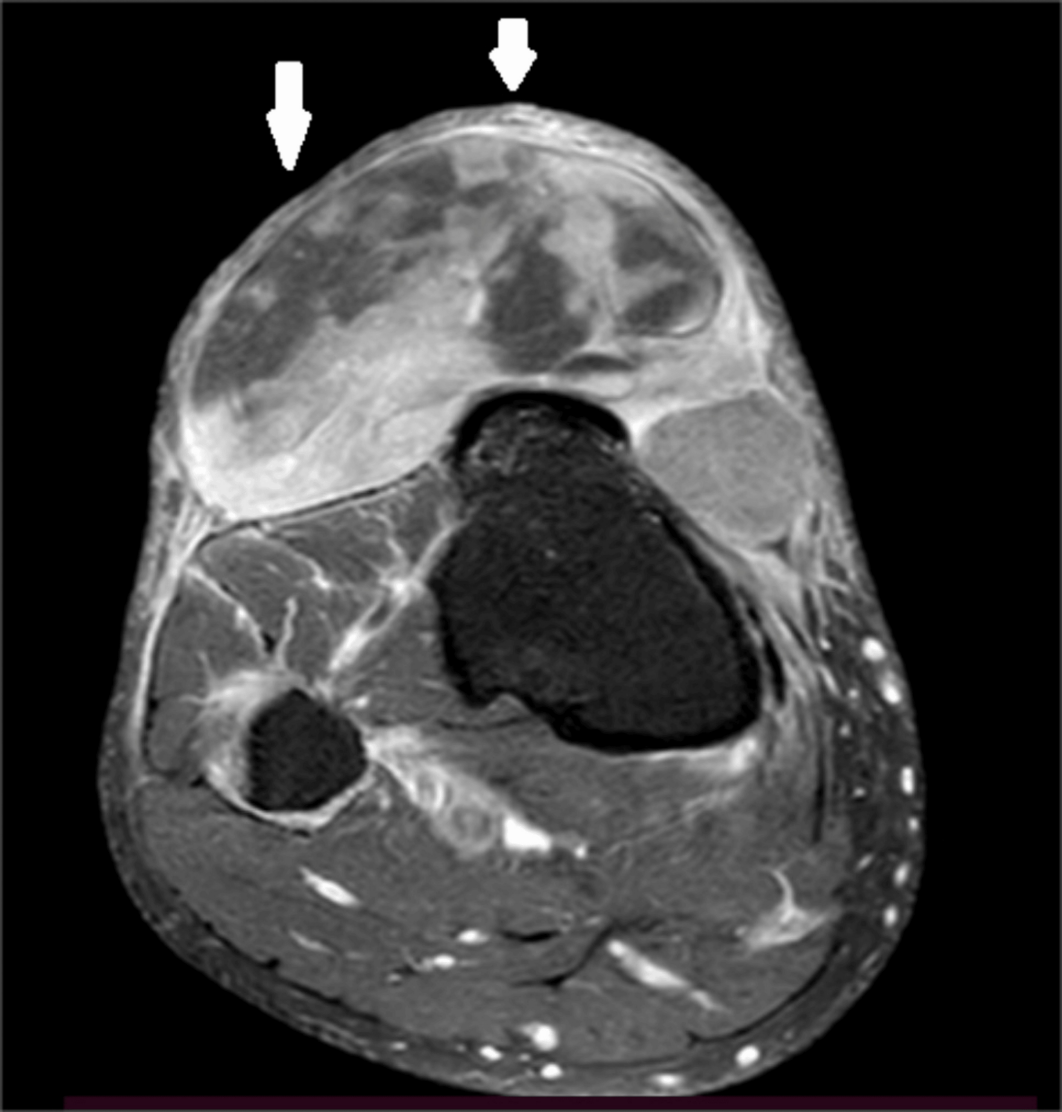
MRI image of the patient; axial section This axial section in the T2 sequence shows the multilobulated appearance of the mass, as well as the necrosis in spontaneous hypersignal, as shown by the arrows.
